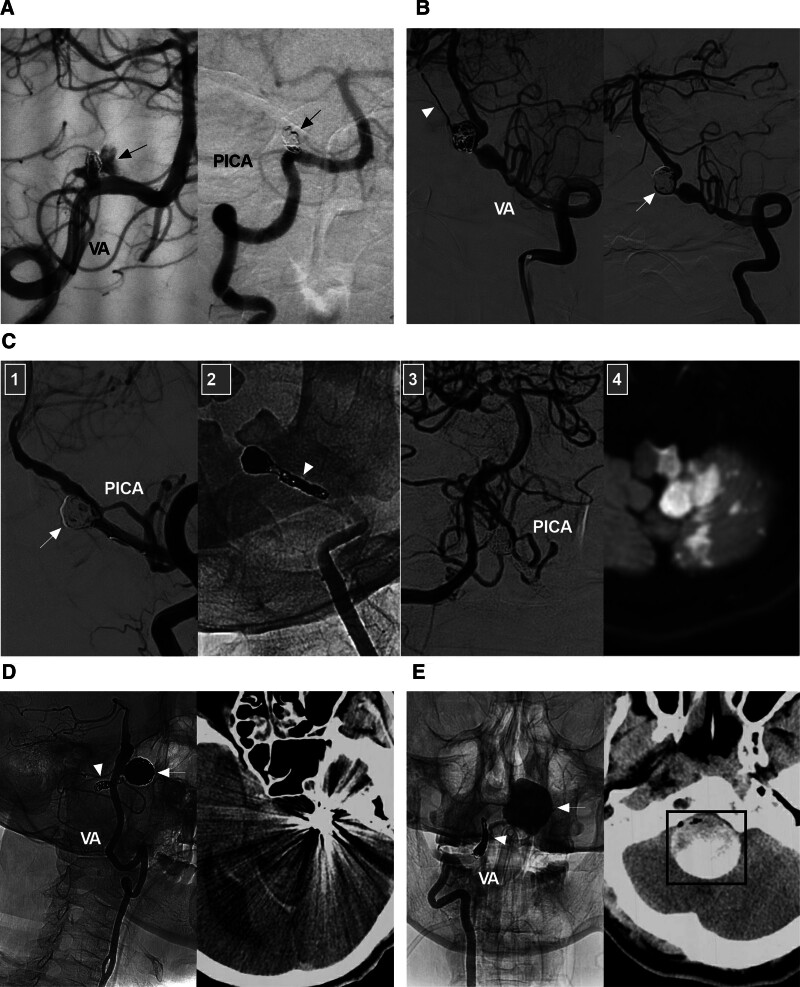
Complications of traditional coiling EVT. (A) Hemorrhagic complications: left panel, DSA image showing an aneurysm at the VA and PICA junction that ruptured during coiling and contrast agent extravasation (arrow); right panel: follow-up DSA image showing complete aneurysm occlusion (arrow). (B) Hemorrhagic complications: left panel: DSA image showing a VA dissecting aneurysm perforated by the microcatheter and a coil loop (arrowhead). right panel: DSA image showing complete coiling of the aneurysm (arrow). (C) Ischemic complications: panel 1: DSA image showing coiling of an aneurysm (arrow) with PICA involvement; panel 2: Unsubtracted DSA image showing occlusion of the VA (arrowhead) proximal to an aneurysm; panel 3: DSA of a contralateral VA showing irrigation of the PICA on the side of the aneurysm by retrograde blood flow; panel 4: postoperative magnetic resonance diffuse sequence image showing acute infarction of the PICA territory. (D) Mild brainstem compression due to a mass effect: left panel: unsubtracted DSA image showing a large VA aneurysm treated by aneurysm trapping (arrow) and proximal PAO (arrowhead); right panel: postoperatively, a patient who experienced hemiplegia shows no hemorrhage on CT imaging. 1 week later, the patient had recovered. (E) Severe brainstem compression due to a mass effect: unsubtracted DSA image showing a large VA aneurysm (arrow) treated by proximal PAO (arrowhead); right panel: 1 day after EVT, the patient experienced respiratory arrest; CT image shows a highly dense aneurysm with a mass effect due to thrombosis (frame); 3 days later, the patient died. CT = computed tomography, DSA = digital subtraction angiography, EVT = endovascular treatment, PAO = parent artery occlusion, PICA = posterior inferior cerebellar artery, VA = vertebral artery.
